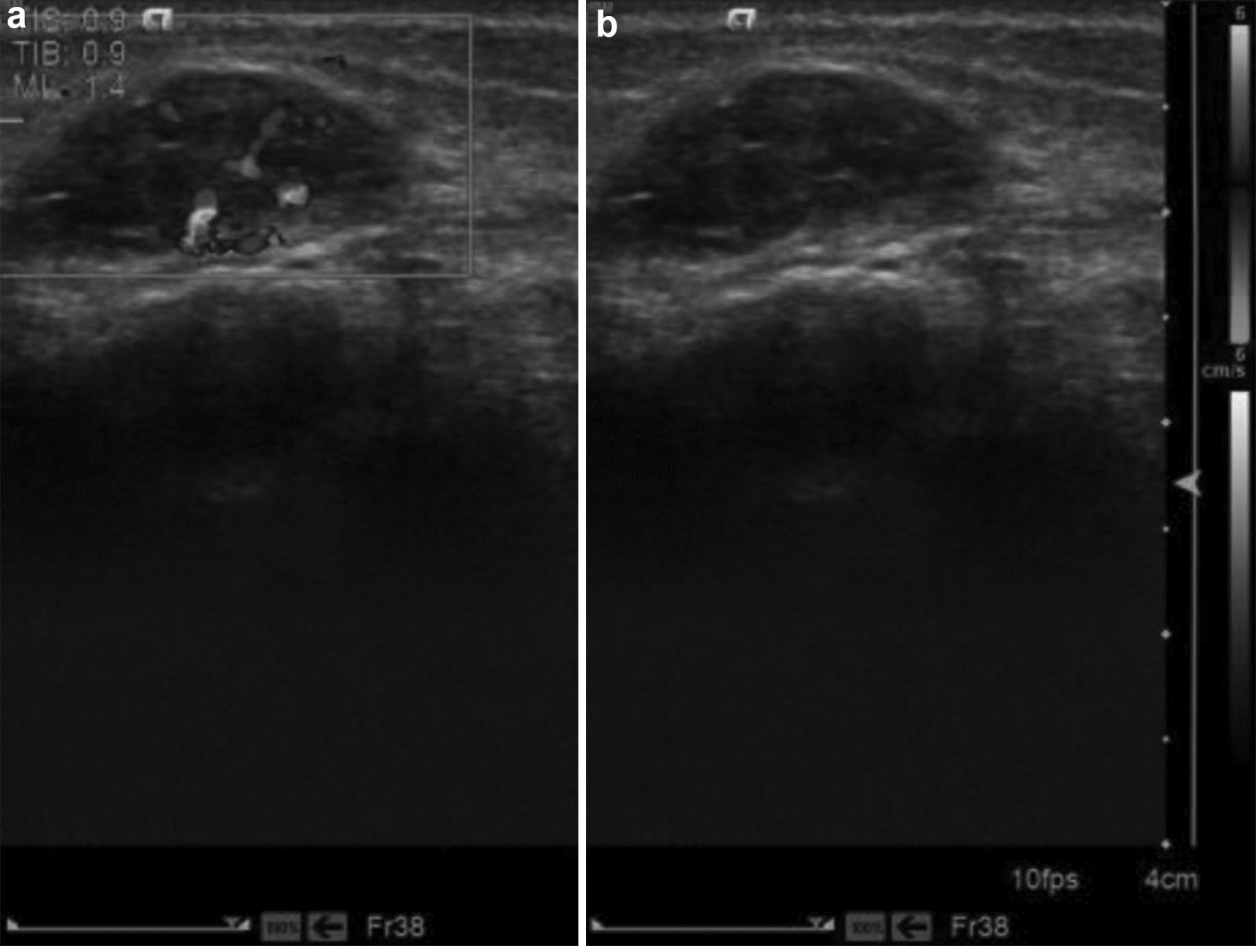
Ultrasound images of female patient (18 years old). a CDFI showed a little intratumoral blood flow signal; b Female, 18 years old, hypoechoic inside the subcutaneous bundle of the right side for lower leg, and muscle gap of the right foot, the figure showed that a little blood flow signal was visible in the muscle bundle of the middle of the calf with clear border
